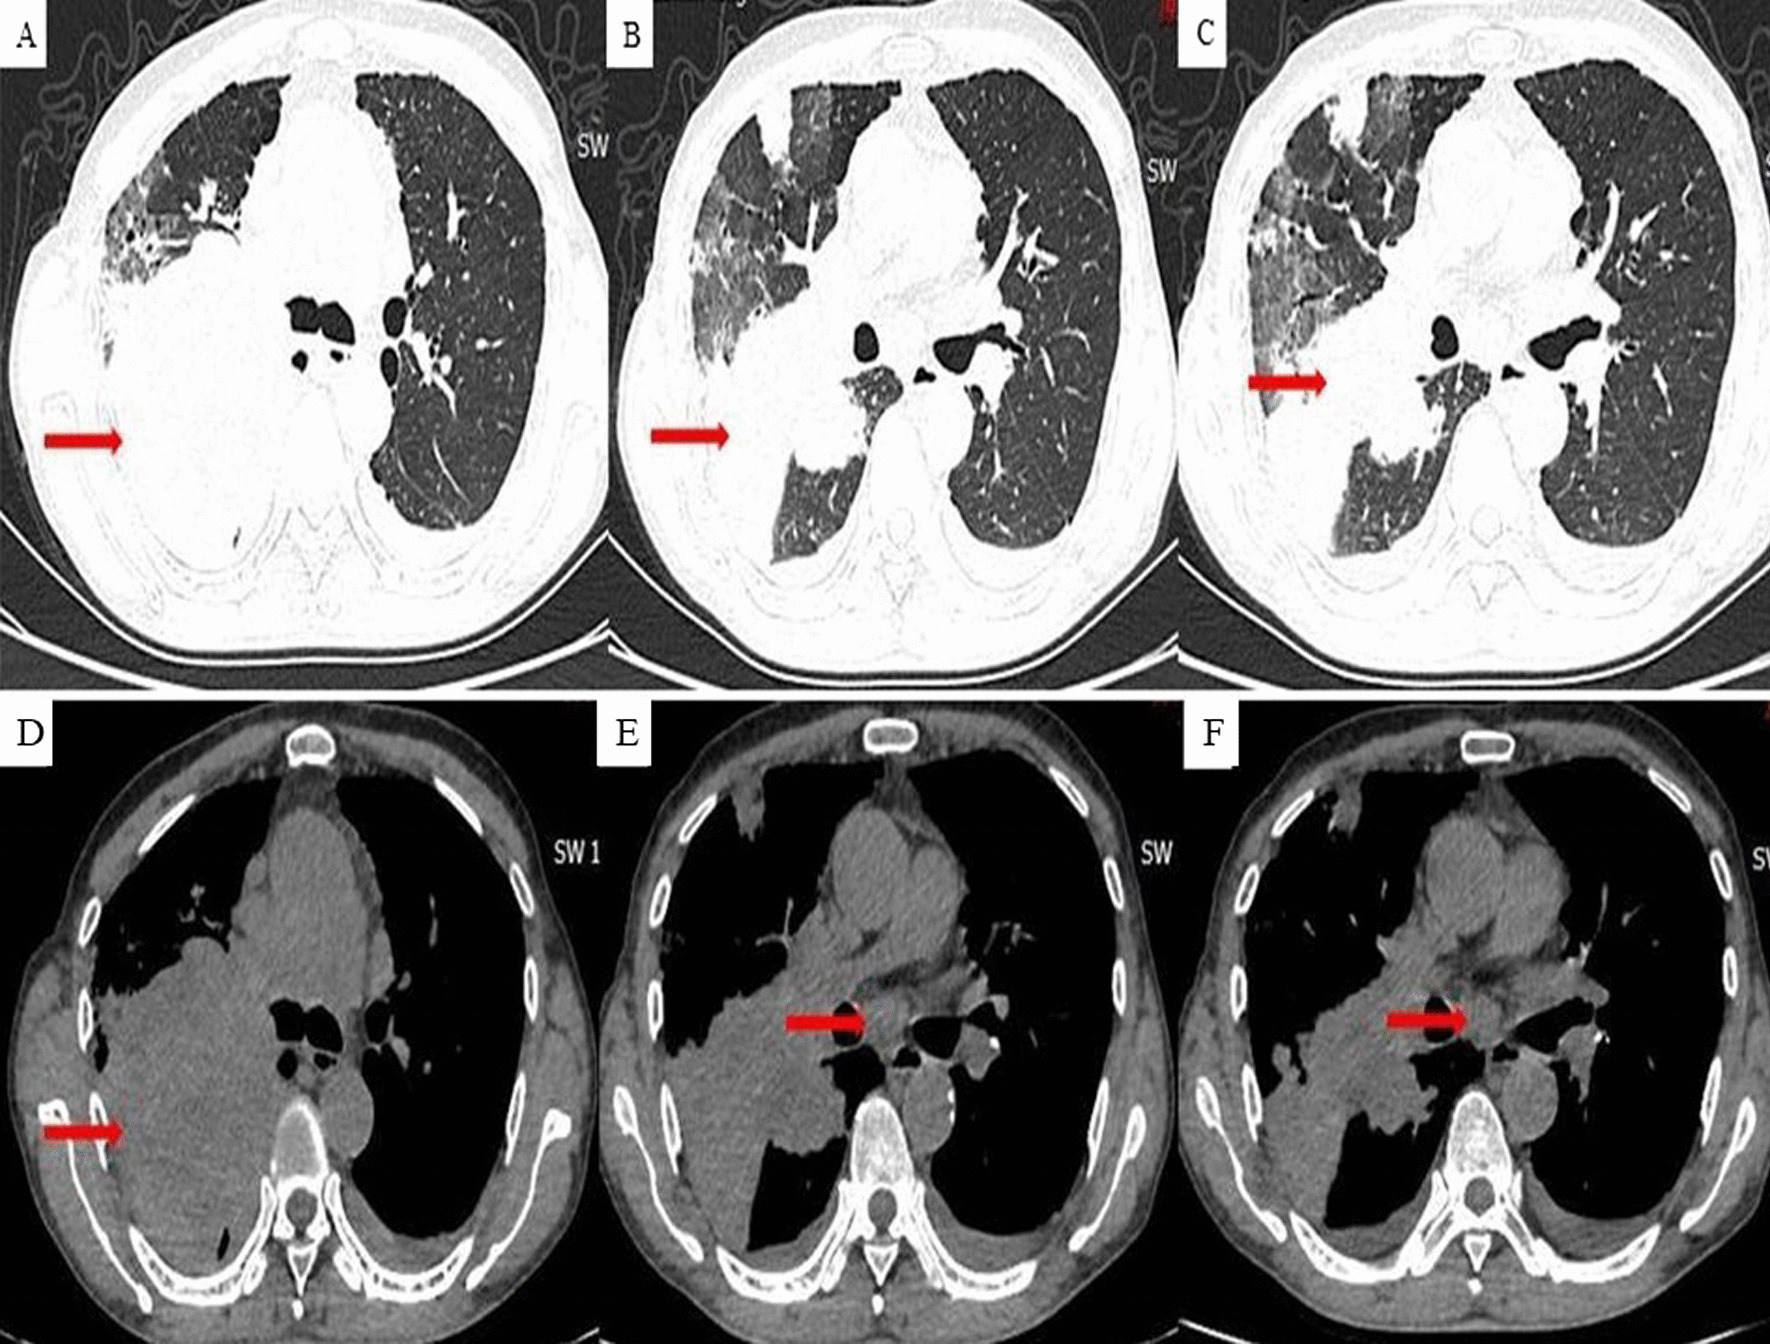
Chest CT image at the initial visit. A mass and mediastinal enlarged lymph node (red arrow) could be seen in the upper lobe of the right lung. a Pulmonary window, trachea bifurcation level, b and c pulmonary window, left upper lobe bronchial branch level. d Mediastinal window, trachea bifurcation level. e and f Mediastinal window, left upper lobe bronchial branch level
